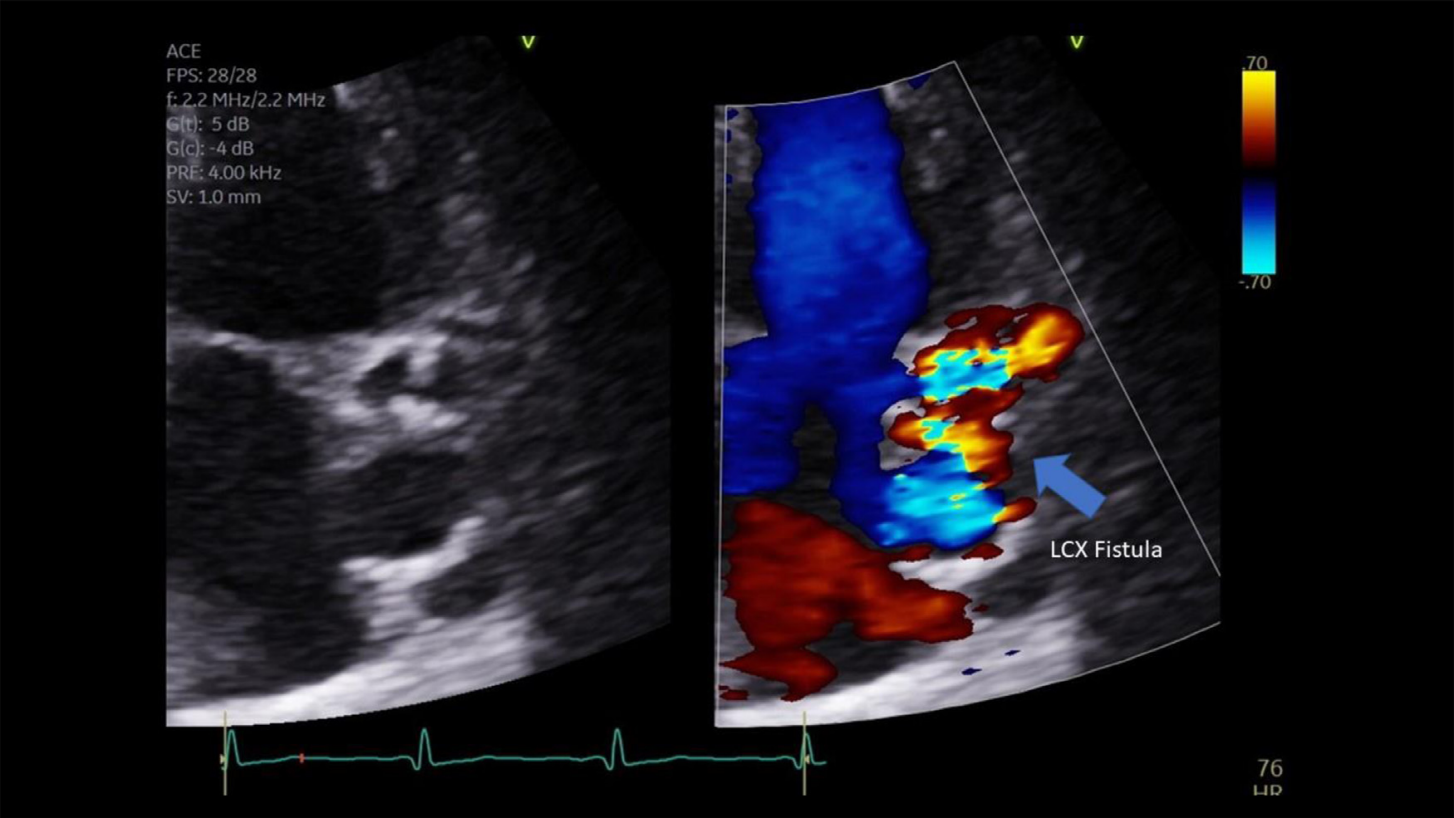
Grayscale and Doppler echocardiogram: the LCX CAF is demonstrated with color aliasing (blue arrow) connecting to the left atrium.
